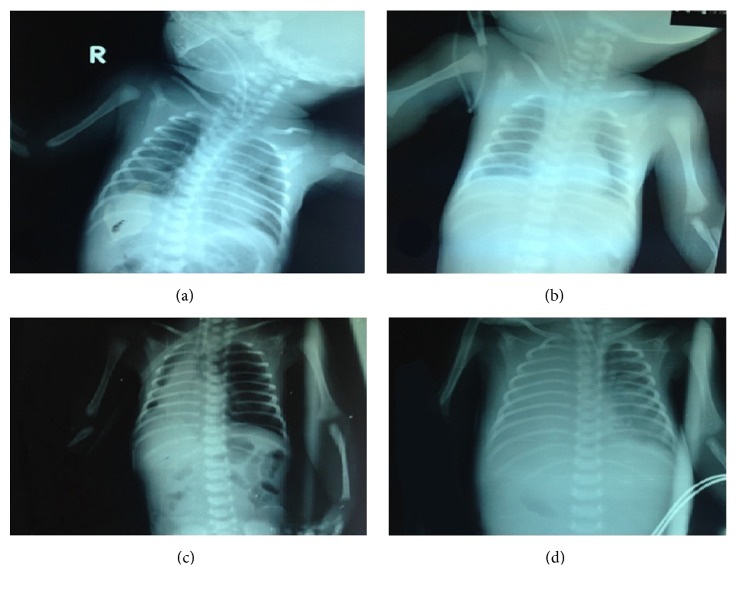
The serial radiographic changes with the development of VAP. A serial of plain chest radiographs along twelve days was obtained in supine anteroposterior position. The first five-day images (a and b) showed normal aeration with average lung volumes, normal mediastinum, and clear costophrenic angles. Also prominent bronchovascular markings are seen, but there is no focal pulmonary lesions. On 6-day image (c), an opacity is developed on the right lower lung zone with air-bronchogram and obliteration of the right costophrenic and cardiophrenic angles, suggesting consolidation. By the twelfth day (d), complete opacification of the right lung is seen causing opaque right hemithorax with mild tracheal deviation to the contralateral left side suggesting development of right pleural effusion. Also small consolidation patches are seen in the left para-cardiac region in middle and lower left lung zones with obliteration of the left cardiac border.
